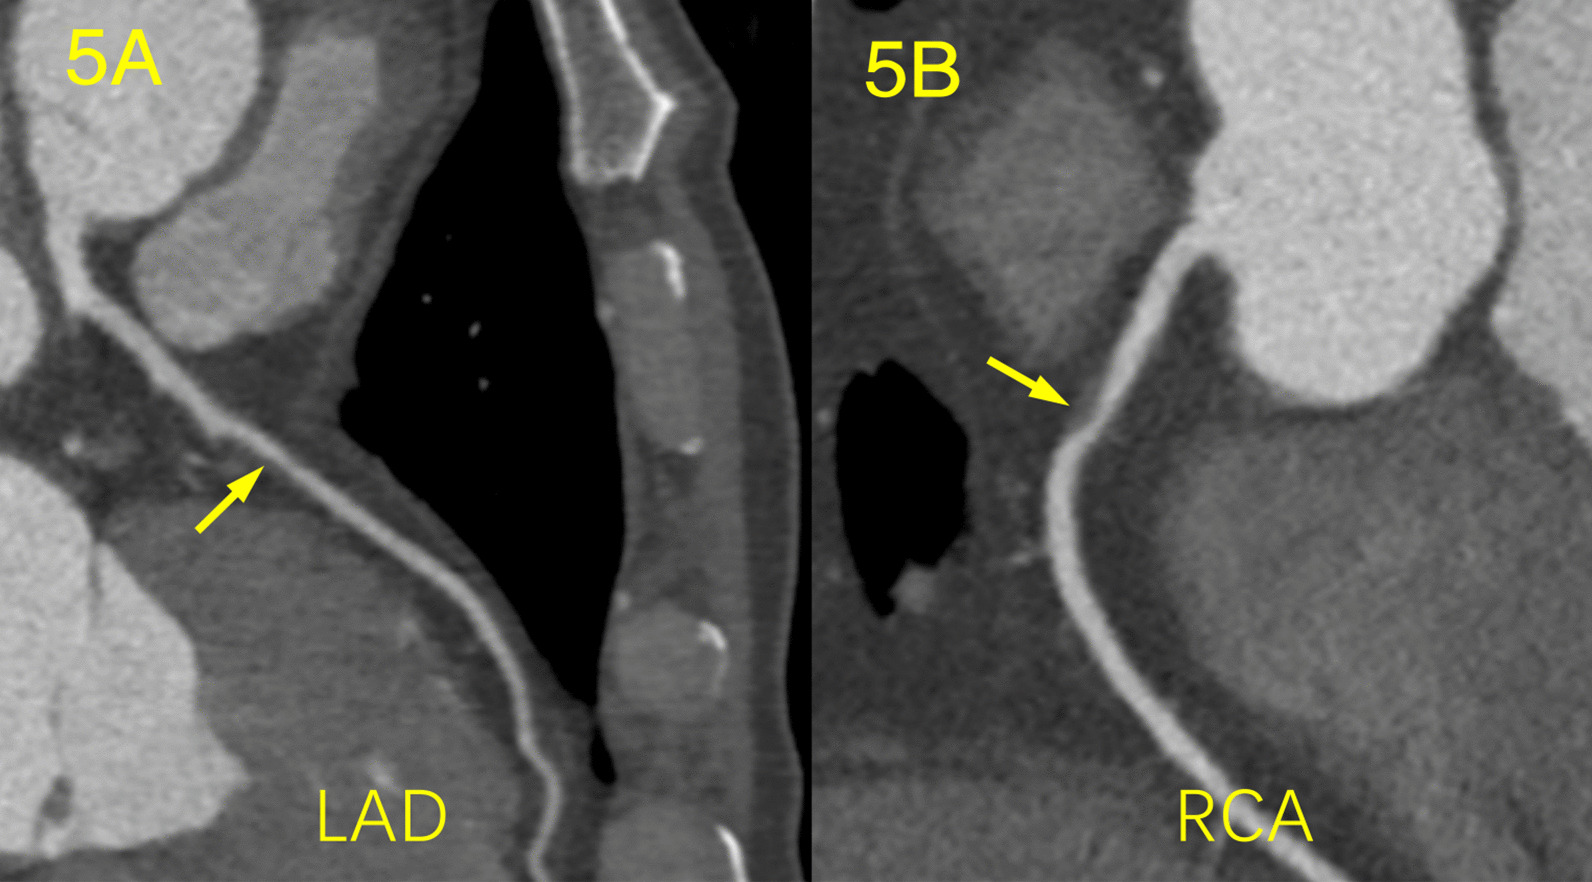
Non-calcified plaques in a 59-year-old man with severe SCH. The patient underwent coronary CTA for chest pain. a Curvature planar reconstruction CT image of the LAD artery shows a non-calcified plaque in the middle segment of the LAD with mild stenosis. b The image of the RCA artery shows a non-calcified plaque in proximal segment of the RCA with severe stenosis
